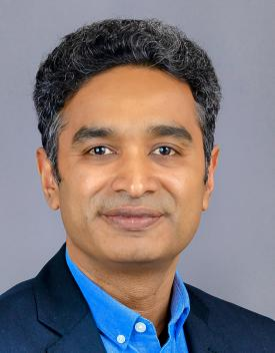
Ashwin Venkateshvaran, PhD, FASE, FESC is Associate Professor of Clinical Physiology and a Senior Staff Scientist at Umeå University, Sweden. His research focuses on advanced cardiac imaging - including echocardiography, cardiac MRI, PET and invasive hemodynamics - to improve early diagnosis, disease phenotyping and risk stratification in heart failure and infiltrative heart disease.
